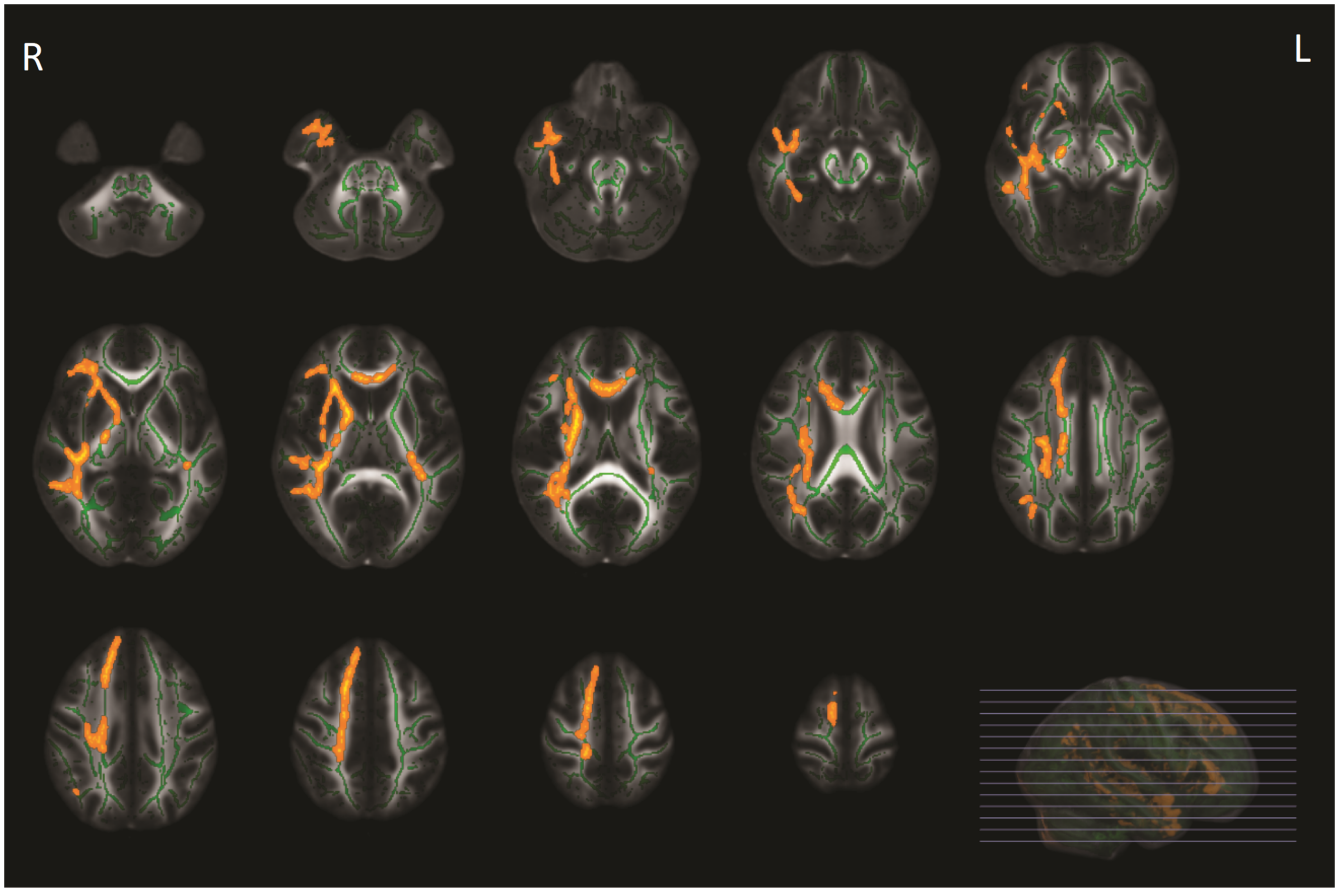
Tract-based spatial statistics (TBSS) analyses of orientation dispersion index (ODI) values in patients with COVID-19 between baseline and follow-up MRI. WM tracts in yellow-orange represent regions with significant ODI values in COVID-19 at baseline compared with ODI values after 3-month follow-up, adjusted for multiple comparisons (*p* < 0.05, family-wise error corrected).
